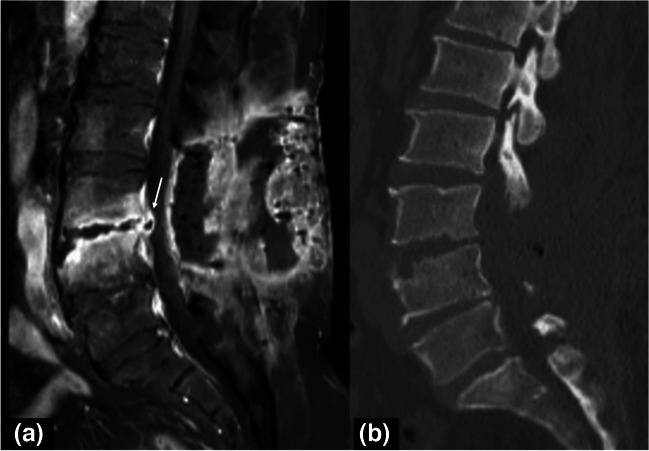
Sagittal fat-suppressed postcontrast T1W MR (a) and corresponding sagittal reformatted CT image in bone window (b) reveal findings of spondylodiscitis in a 65-year-old women with a recent history of operation on herniated L3-4 intervertebral disk who presented with back pain and discharge at the operation site. Note the large soft tissue abscess with peripheral rim enhancement extending all the way between skin to posterior epidural space at the level of L3-L4 vertebral bodies. There is also an anterior epidural abscess (arrow) and marked enhancement in L3-L4 endplates. CT image (b) better demonstrates end-plate irregularities and bony erosions
